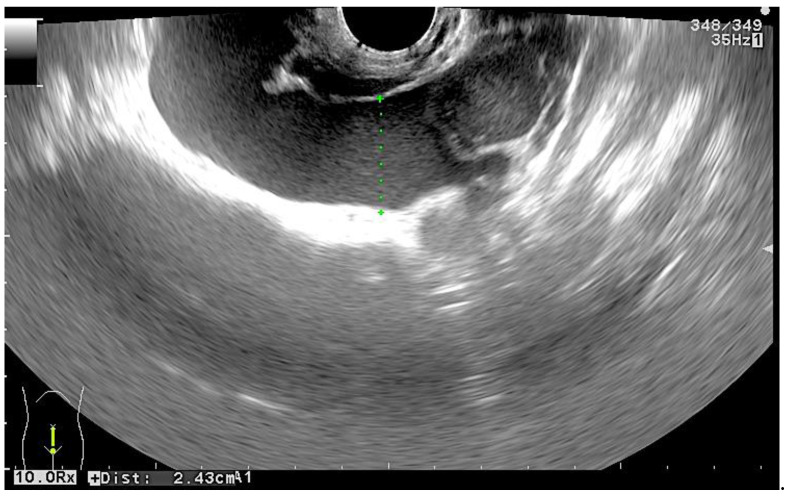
Transvaginal ultrasonography image showing free intraperitoneal fluid in pelvis.
